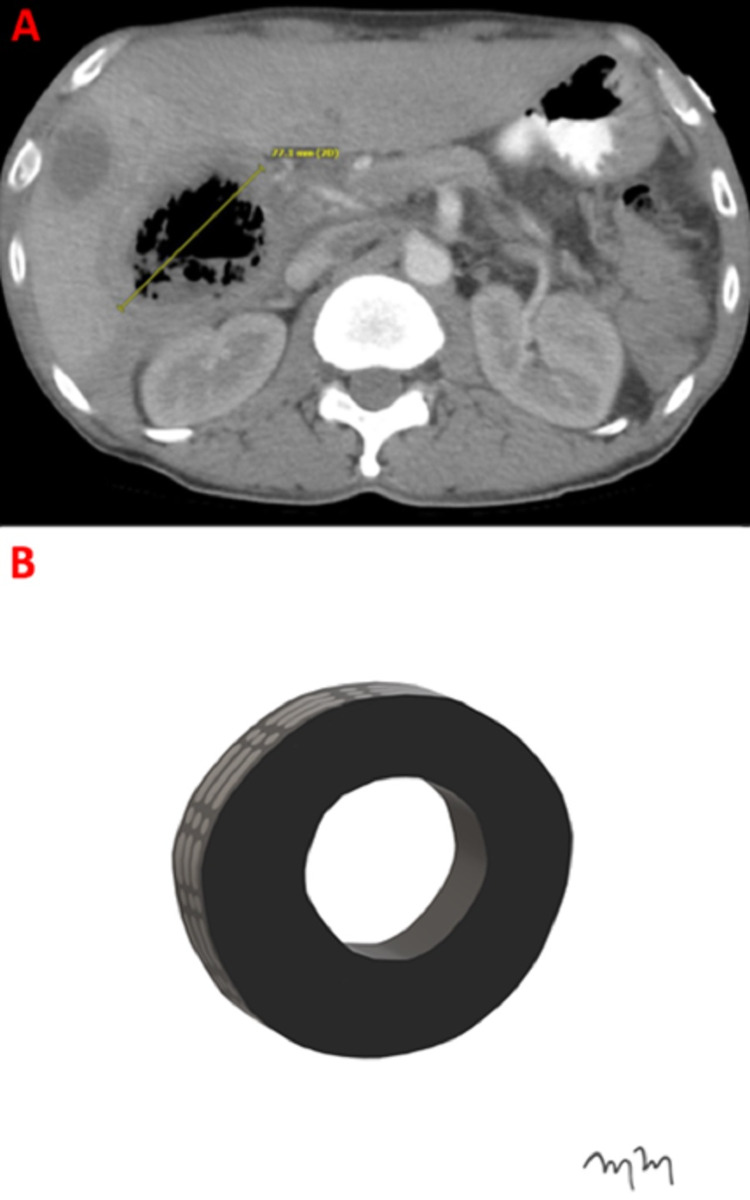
Intrahepatic Abscess Representing an Inflated Tire An axial computed tomography with intravenous contrast at the level of the inferior right hepatic lobe demonstrates a 77.1-millimeter abscess within hepatic segment six (A). An artist rendition image shows an inflated tire, representing the intrahepatic abscess (B).
